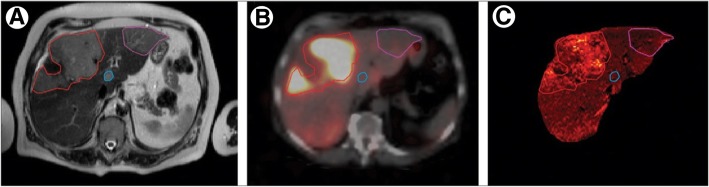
Intrahepatic visualisation of ^166^Ho-microspheres after SIRT. T2-weighted MRI of the liver in a patient with several ocular melanoma liver metastases, outlined by coloured regions of interest (a). After SIRT, the distribution of ^166^Ho-PLLA-microspheres within the liver was visualized by single-photon-emission CT (b) and R2-weighted MRI (c). Reprinted from The Lancet Oncology, Vol. 13, Smits et al., Holmium-166 SIRT in patients with unresectable, chemorefractory liver metastases (HEPAR trial): a phase 1, dose-escalation study, 1025–1034, Copyright 2012, with permission from Elsevier
